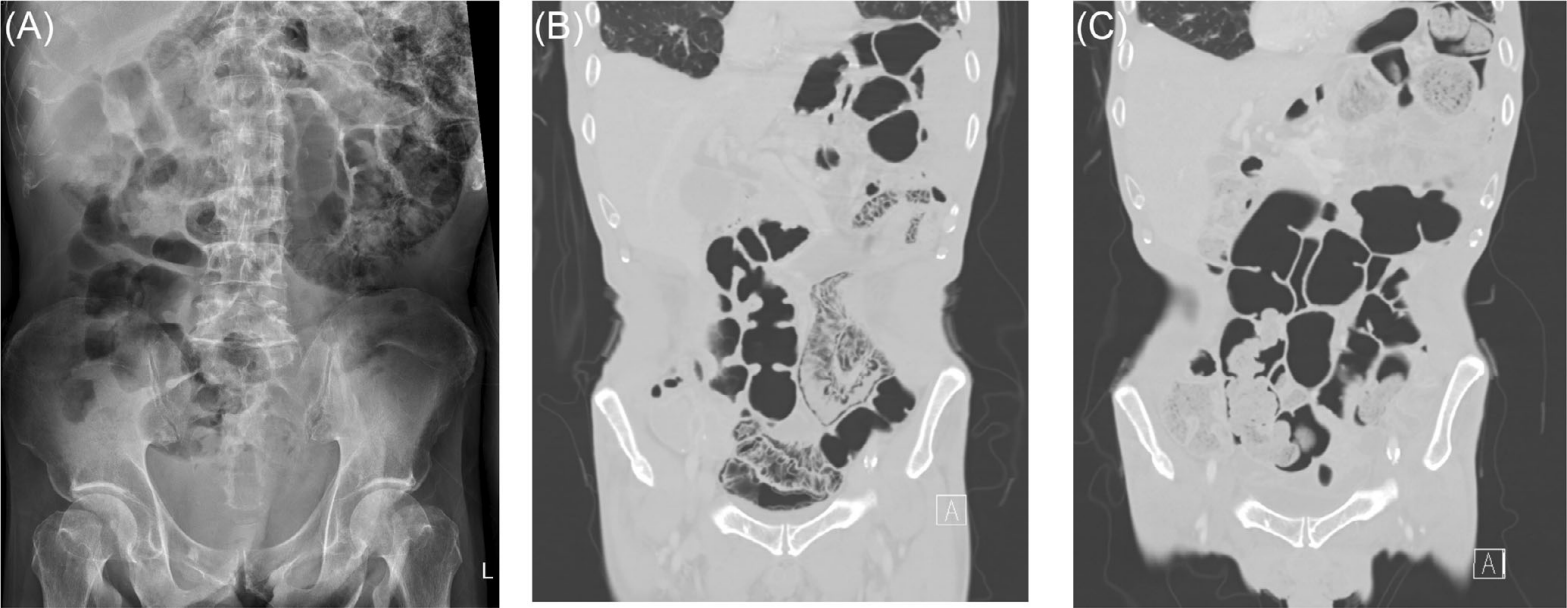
(A) The abdominal radiograph and (B) the coronal‐view abdominal computed tomography (CT) image showing the jejuno‐ileal pneumatosis intestinalis, which later completely resolved as revealed by (C) the follow‐up CT image 6 months later.
